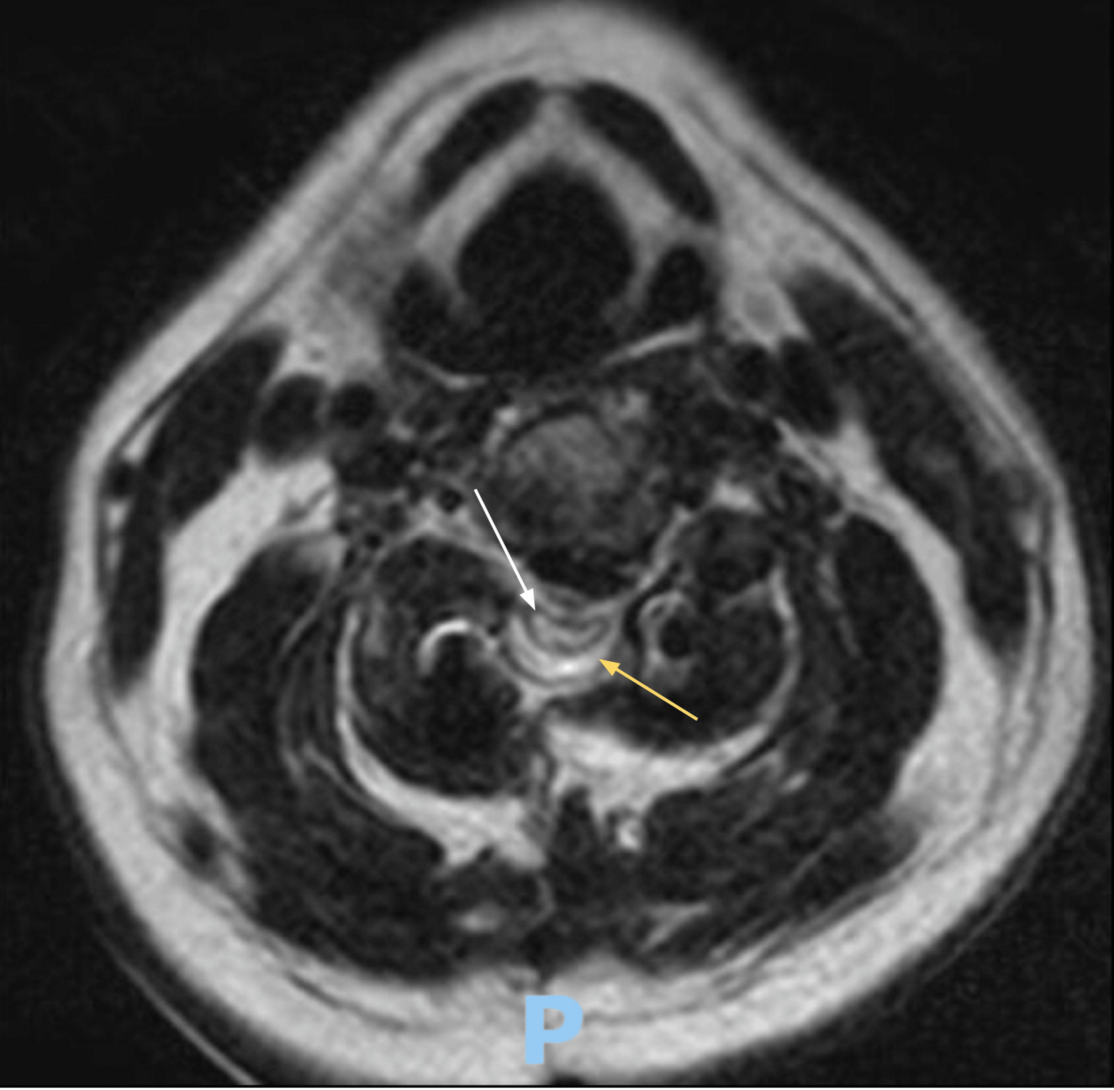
Axial view of the postoperative cervical T2-weighted MRI at the level of C3-C4. An adequate CSF signal around the spinal cord is seen, which suggests adequate decompression (yellow arrow). An intramedullary T2-weighted hyperintensity is noted in postoperative imaging (white arrow). P: Posterior
